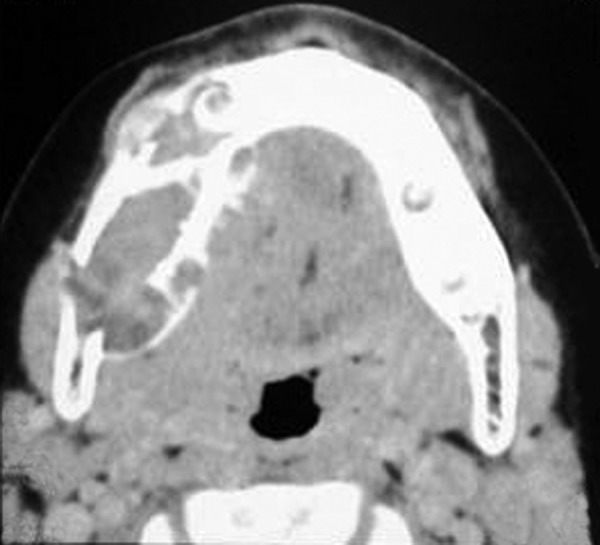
CT axial section shows multilocular expansile lytic lesion in body of mandible right side with significant enhancing soft tissue matrix
